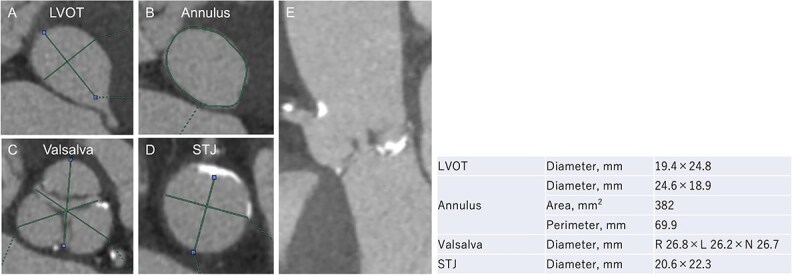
Evaluation of the aortic valve complex using contrast-enhanced CT shows axial views (A–D) and a curved multi-planar reconstruction (E) with a narrowed sinotubular junction and small calcifications (LVOT: left ventricular outflow tract; STJ: sinotubular junction).
